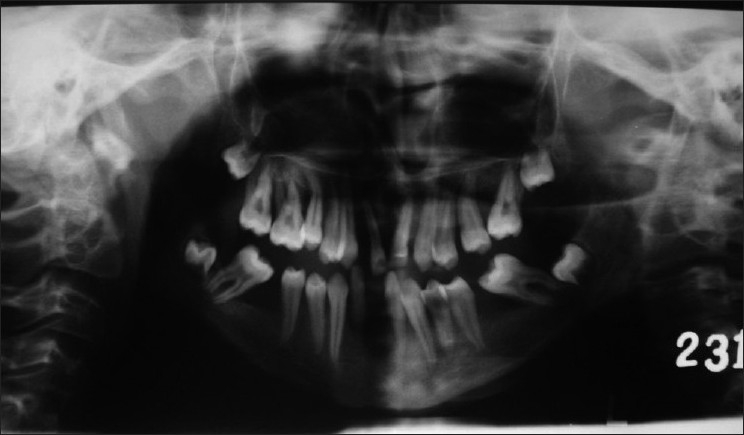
Panoramic radiograph. Note severe alveolar bone loss in relation to the permanent teeth
